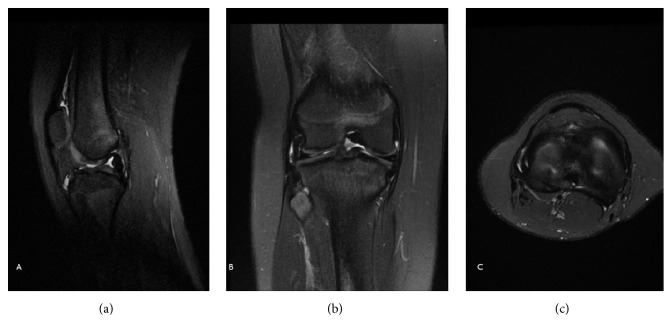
MRI (sagittal (a), coronal (b), and transverse (c) views) of the right knee of a 15-year-old female patient shows complete agenesis of the ACL and a normal PCL.
